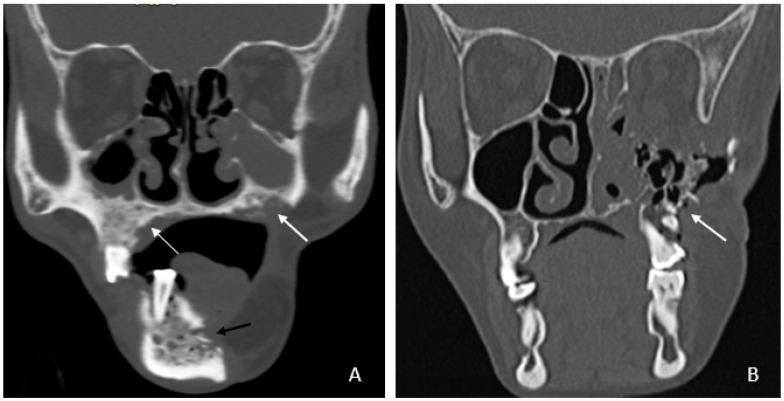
(A) Coronal CT image showing involvement of bilateral upper alveolus (white arrow) and mandible with areas of cortical erosion, trabecular thickening, and sclerosis (black arrow). (B) Coronal CT image showing extensive ORN of the left maxilla—seen as destruction and collapse (arrow).
